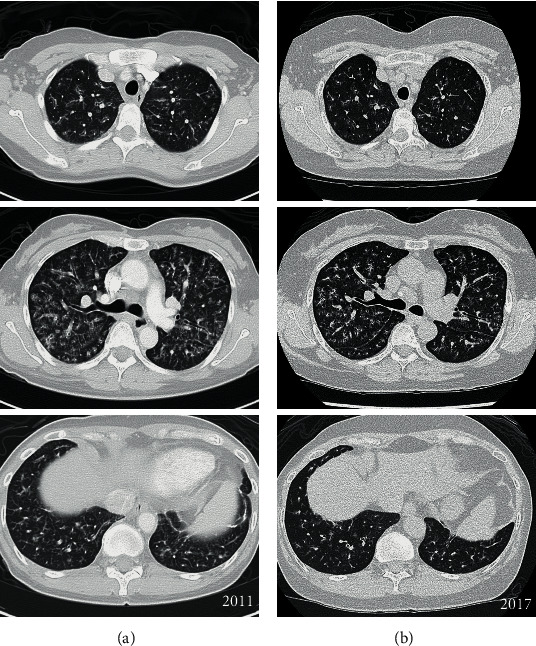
Selected axial CT images of the initial scan (a) and 6 years later (b) of LIP. There are diffuse peribronchovascular thickening and ground glass changes with tiny cysts along the bronchovascular bundles in both lungs.
